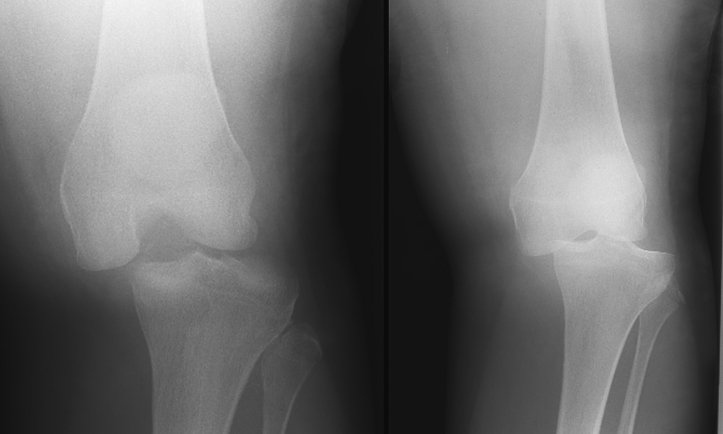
AP radiographs of both patients with tibial plateau fractures with complete dislocation and total loss of joint congruity. These fractures are anteromedial and therefore harder to visualize on AP views.
